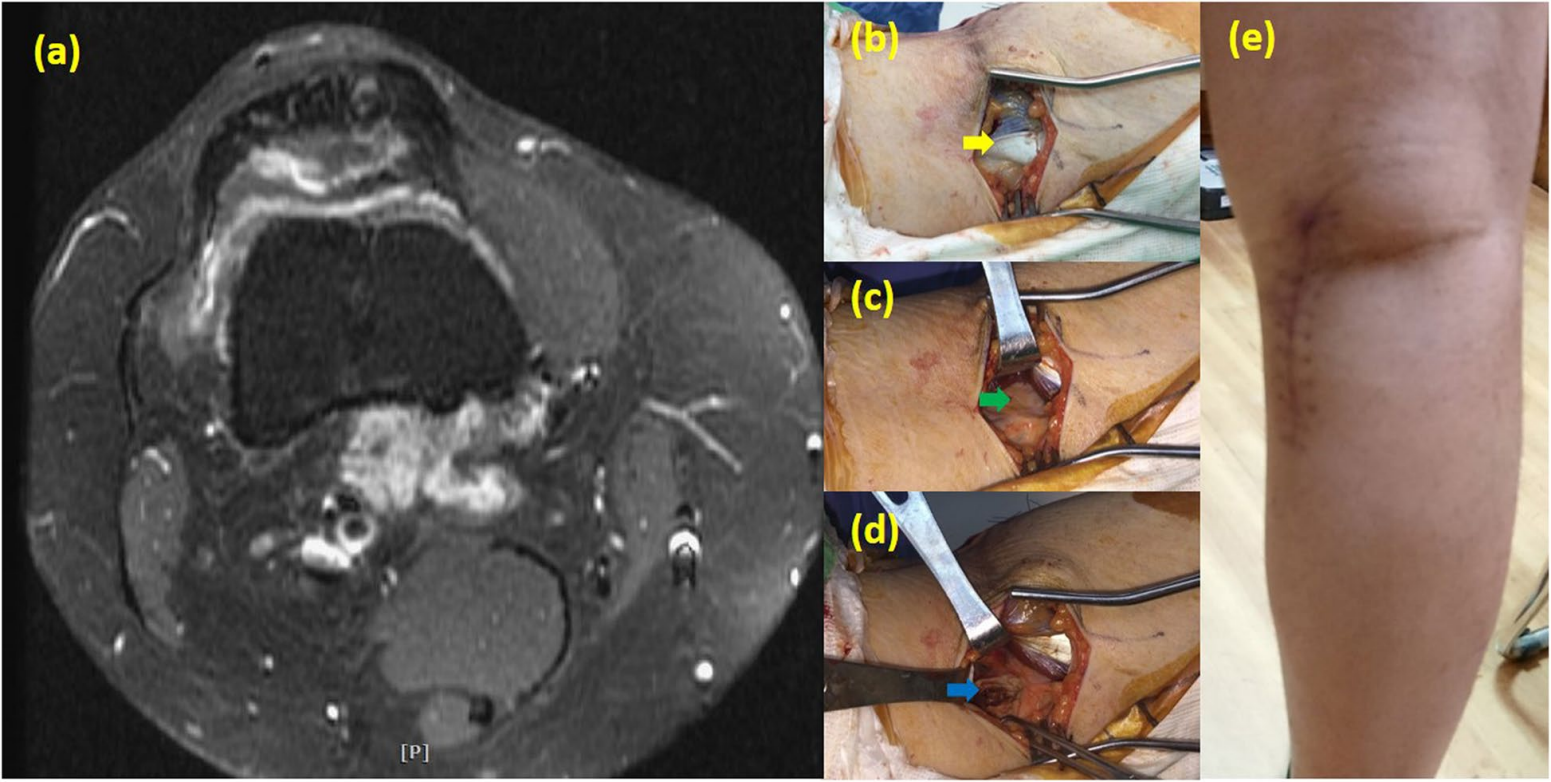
44-year-old woman suffered from DPVNS of right knee. (b-d) PM approach. (a) MRI of proton density with fat saturation sequences axial view showed the PVNS tumor located at anterior and posterior-medial side. (b) Place the incision over the medial head of the gastrocnemius. Yellow arrow: Gastrocnemius muscle medial head. (c) After retracting the medial gastrocnemius medially, the posterior-medial capsule was exposed (see green arrow). (d) After opening the capsule, PVNS tumor were seen deep to the joint capsule. Blue arrow: PVNS tumor. (e) Postoperative image of the operative knee with PM approach.
